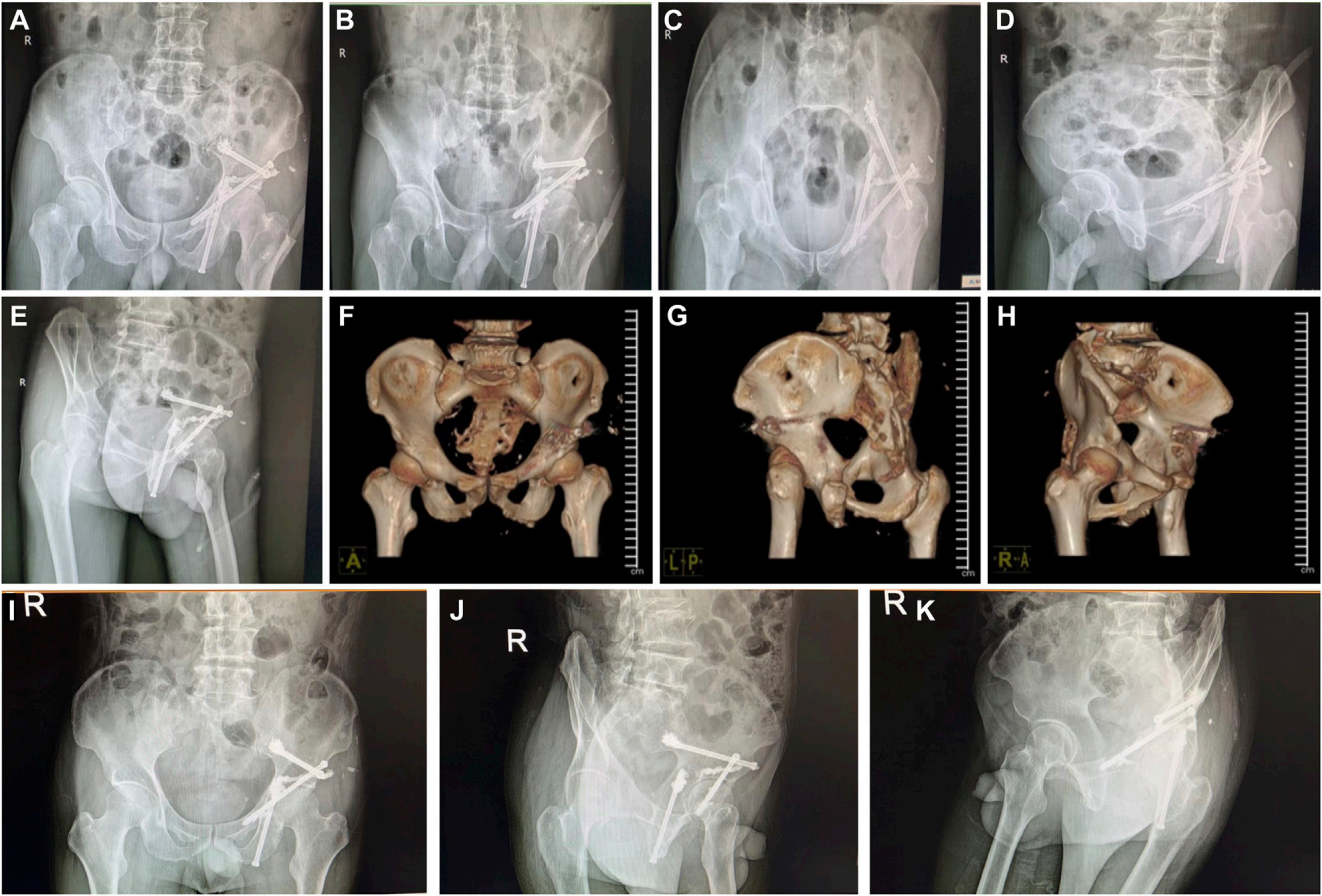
Postoperative review of X-ray radiographs and CT 3D reconstruction, and the postoperative follow-up X-ray images of the patient. (A–E) Different angles of X-ray radiographs on the pelvis to evaluate the operation (F–H) CT 3D reconstruction of the pelvis to assess the operation. (I–K) Different angles of X-ray radiographs on the pelvis to evaluate the operative area in the postoperative follow-up patient.
